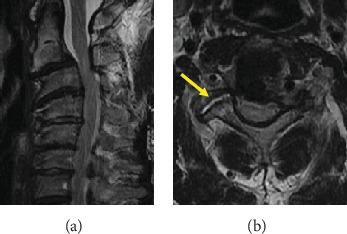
Preoperative MRI images. (a) Preoperative MRI images showed spinal cord compression at C2-3 level with spinal edema. (b) Axial MRI images of C2-3 right facet joint indicate an articular edema.
